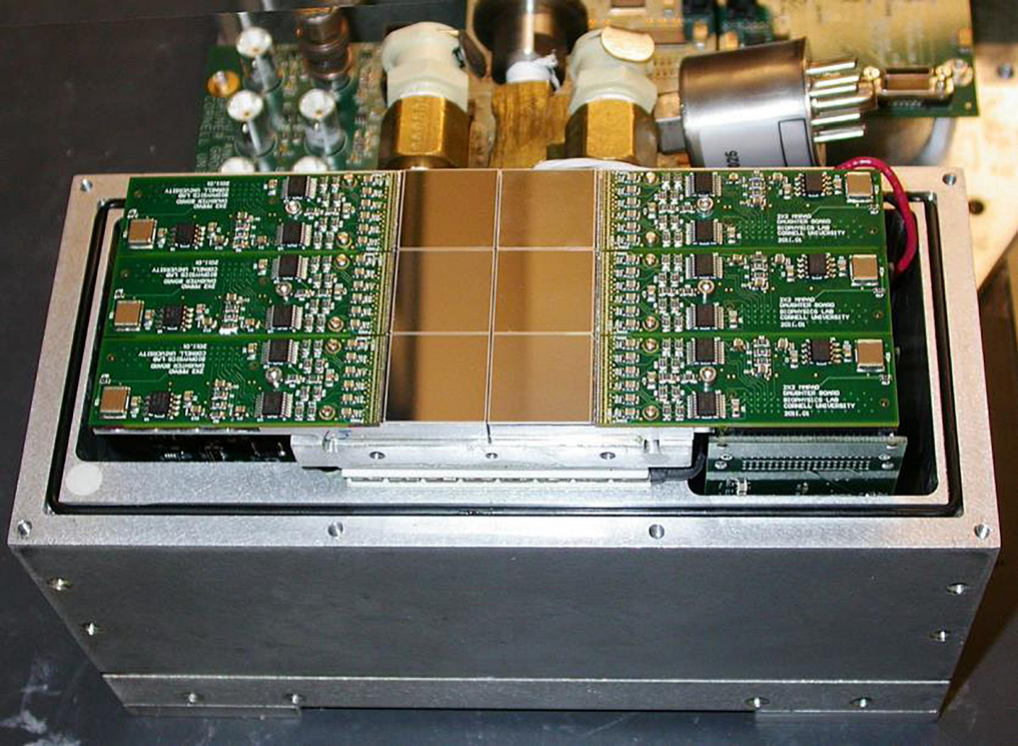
The MM-PAD is shown with the vacuum cover and x-ray window removed. The 2 × 3 arrangement of detector modules are the brownish squares in the center. Each module consists of 128 × 128 square pixels, where each pixel is 150 *μ*m of a side. Each module is roughly 2 cm × 2 cm in size. There is a 5 pixel wide (0.75 mm) inactive area between adjacent modules. (This photo is of an MM-PAD with Si, instead of CdTe sensors; otherwise, the two types of MM-PADs look identical.)
